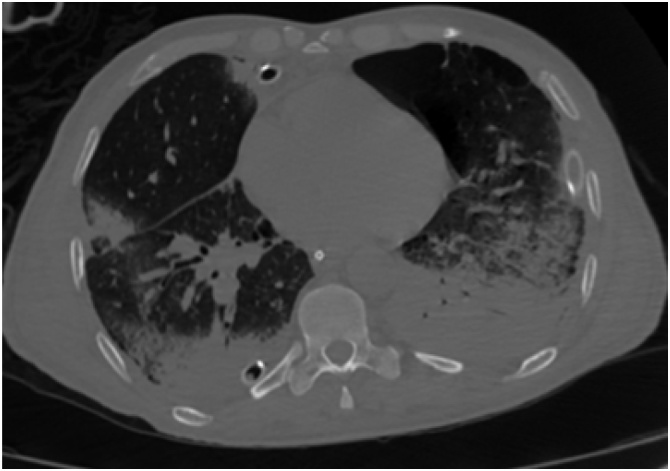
Thorax CT without contrast demonstrating a bilateral pulmonary consolidation despite pulmonary expansion.
